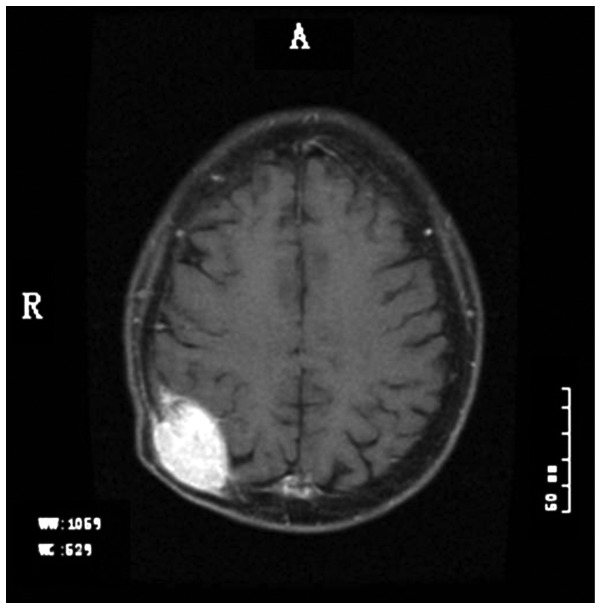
Case 2. Mass destruction and skull defect in the right parietal bone by magnetic resonance imaging.
